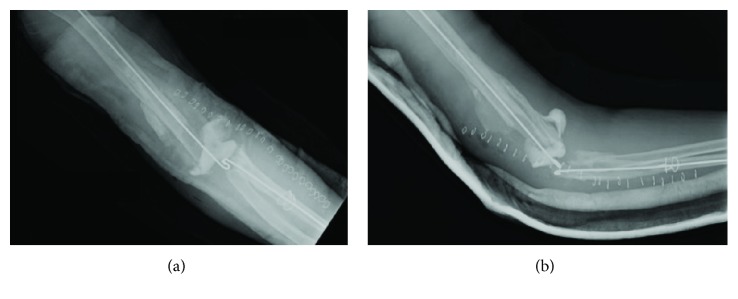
(a) AP and (b) lateral radiographs obtained following resection arthroplasty with placement of an amphotericin, vancomycin, and tobramycin-impregnated cement spacer.
